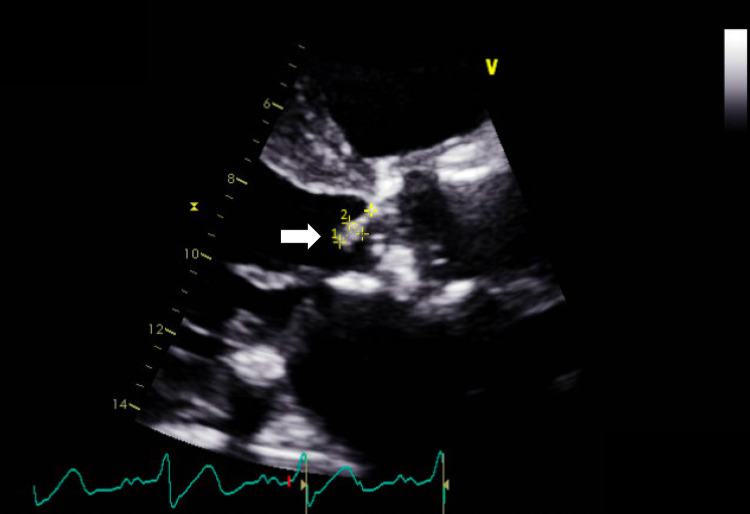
Transthoracic echocardiogram parasternal long axis aortic valve focused view An approximately 1.2 x 0.4 centimeter echogenic density (arrow) is attached to the aortic valve towards the left ventricular outflow tract.
